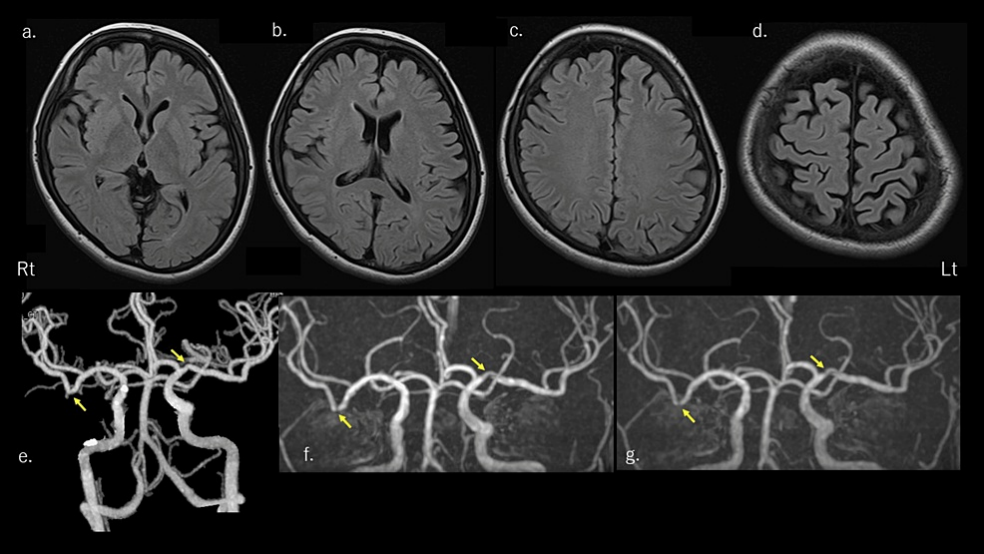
Brain magnetic resonance imaging (MRI), computed tomography angiography (CTA), and magnetic resonance angiography (MRA) The upper row images show brain MRI fluid-attenuated inversion recovery images performed at the time of initial diagnosis (day 3 of onset). (a-d): There were no abnormal findings. The lower row images show head CTA at the initial visit (day three onset) (e) and head MRA at days 35 and 84 onset (f and g). (e): There was no obvious stenosis in the main trunk artery, but there was slight stenosis in the left middle cerebral artery (MCA) M1 and right MCA M2 segment. (f, g): No obvious changes were observed on head MRA at 35 and 84 days of onset. Both had slight stenosis of the left MCA M1 and right MCA M2 segments. This was thought to be due to flexure or mild sclerotic changes.
